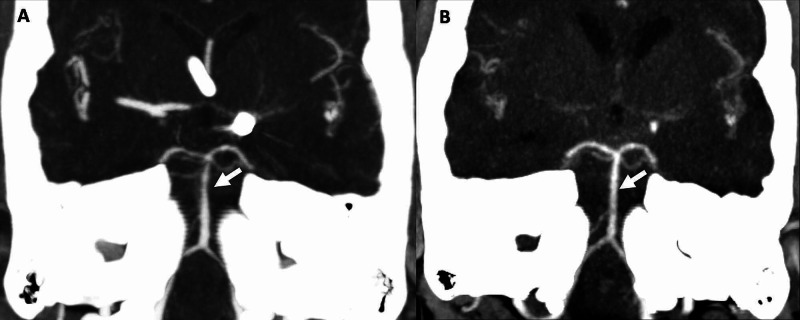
CT angiogram head Coronal MIP CT angiogram image (Figure 2A) shows diffuse narrowing of the distal basilar artery (arrow). Follow up CT angiogram coronal MIP image (Figure 2B) demonstrates resolution of the basilar vasospasm (arrow).
